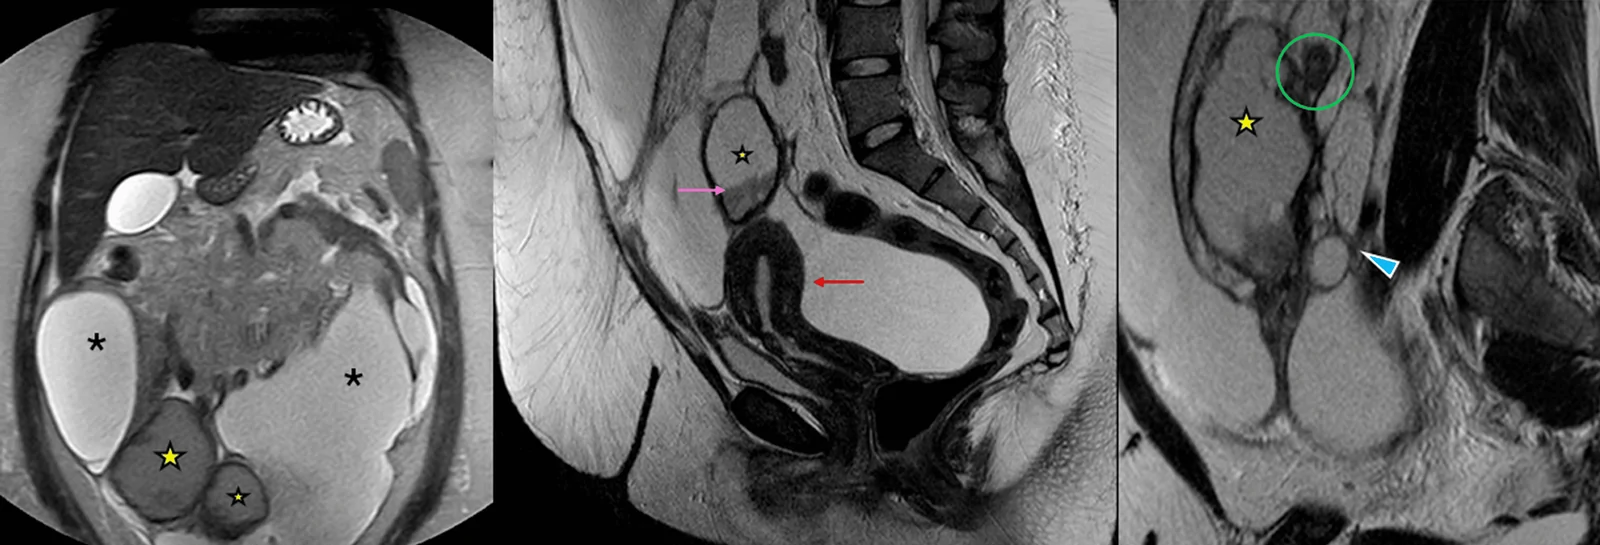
Abdomen and pelvis MRI (coronal and sagittal T2) The lesion shows high T2 signal intensity with a shading sign (yellow stars) and thick walls. Ascites is present in the inframesocolic compartment (asterisks). A fluid-fluid level is also observed (pink arrow). The uterus is unremarkable (red arrow). The bilobed lesion is adjacent to the anterosuperior margin of the right ovary (blue arrowhead) and the distal ileum (green circle). MRI: magnetic resonance imaging
